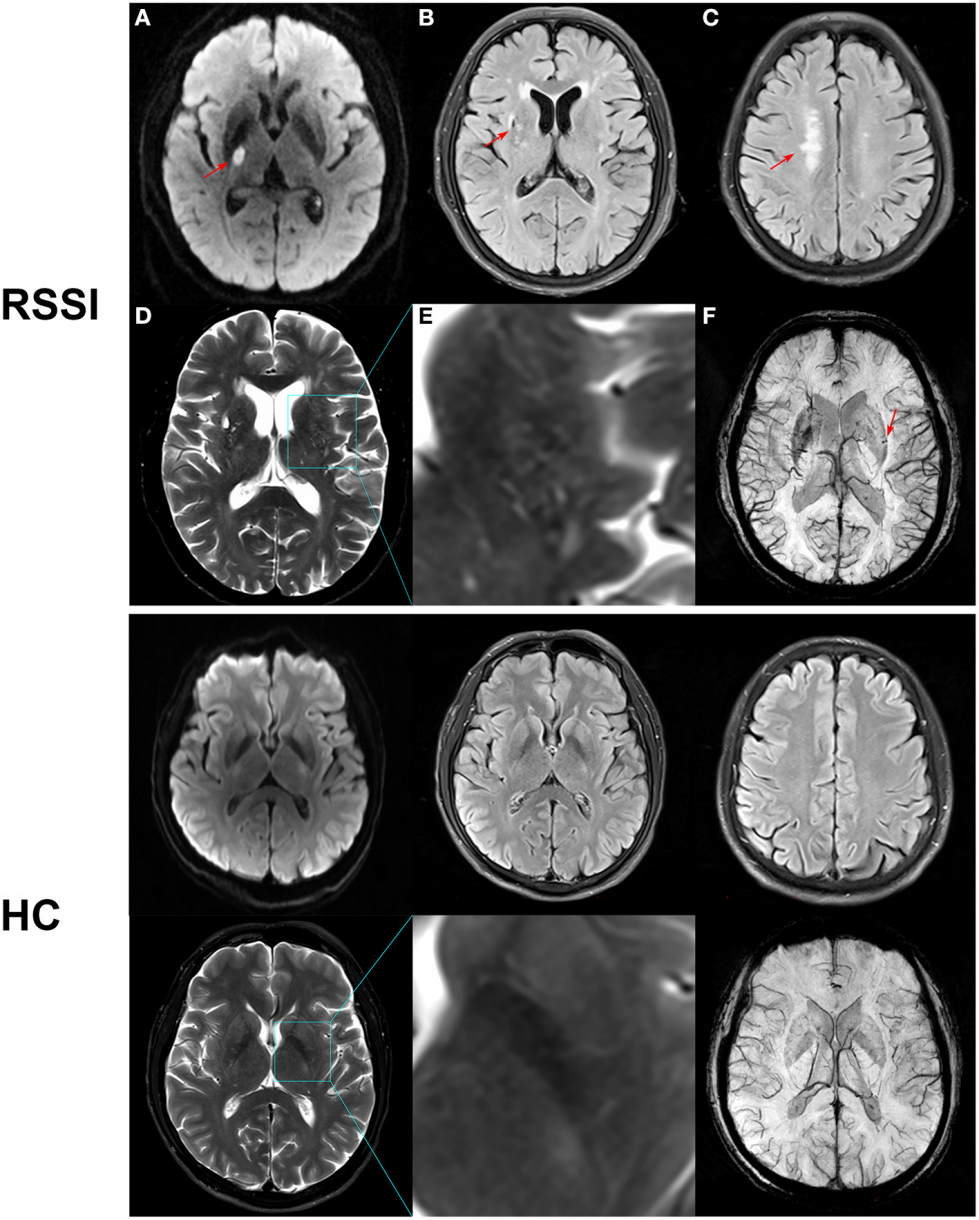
Representative MR images of recent subcortical infarction (RSSI) and healthy control (HC). Upper row: (A) DWI showed an acute single subcortical infarction in the right basal ganglia (arrow). (B) FLAIR showed a chronic lacune in the right basal ganglia (arrow). (C) FLAIR showed deep white matter lesions (arrow) with a Fazekas score of 2. (D) T2WI showed moderate enlarged perivascular space in the basal ganglia. (E) Magnified image of the outlined region indicated in (D). (F) SWI showed cerebral microbleeds in the left basal ganglia. This patient had a total CSVD score of 4. Bottom row: A 55-year-old healthy control showed normal MR images with a total CSVD score of 0.
